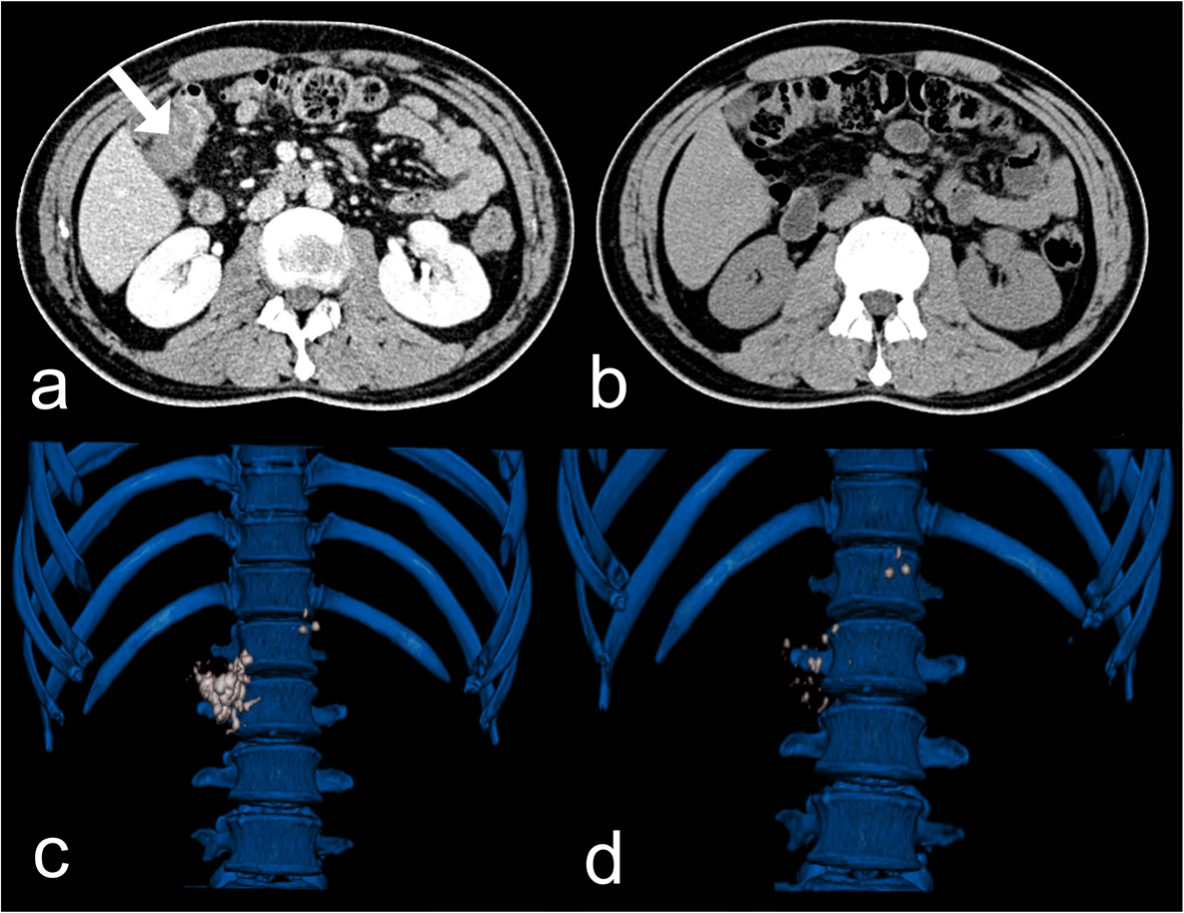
a The contrast-enhanced computerized tomography (CT) scan shows there is a colonic space occupying lesion (white arrow) in the hepatic flexure of colon. b CT scan shows the colonic hematoma disappeared at the three-month follow up. c The three-dimensional CT reconstruction of abdominal shows the pancreas with radiopaque stones in the whole pancreatic body before pancreatic extracorporeal shock wave lithotripsy (P-ESWL). d Three-dimensional reconstruction of CT scan shows the pancreatic stones has decreased significantly at the three-month follow up
